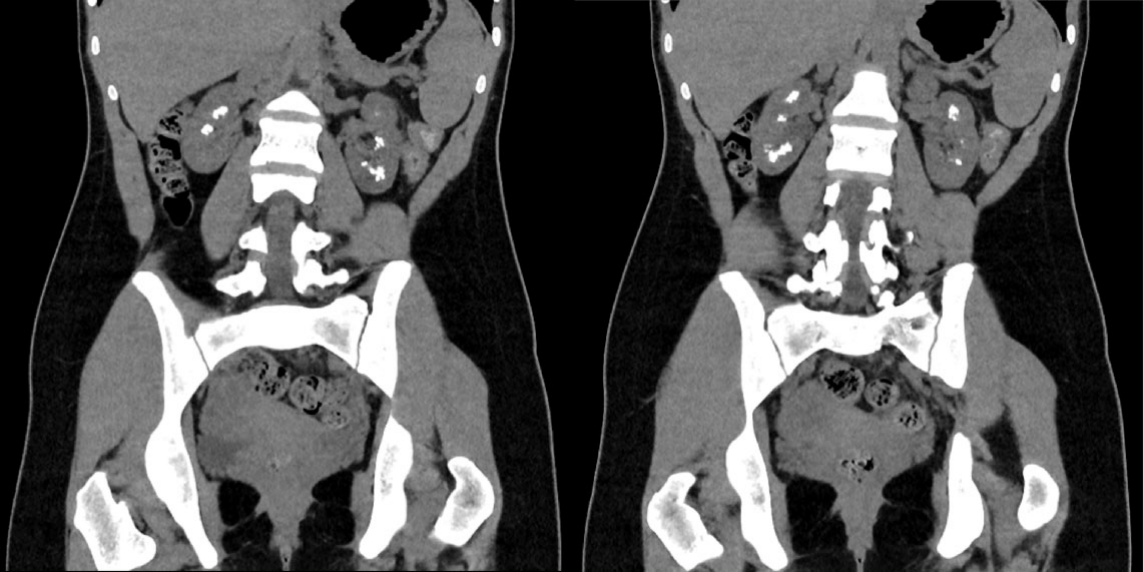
Computed tomography of the kidneys, ureters and bladder showing extensive bilateral medullary nephrocalcinosis.
